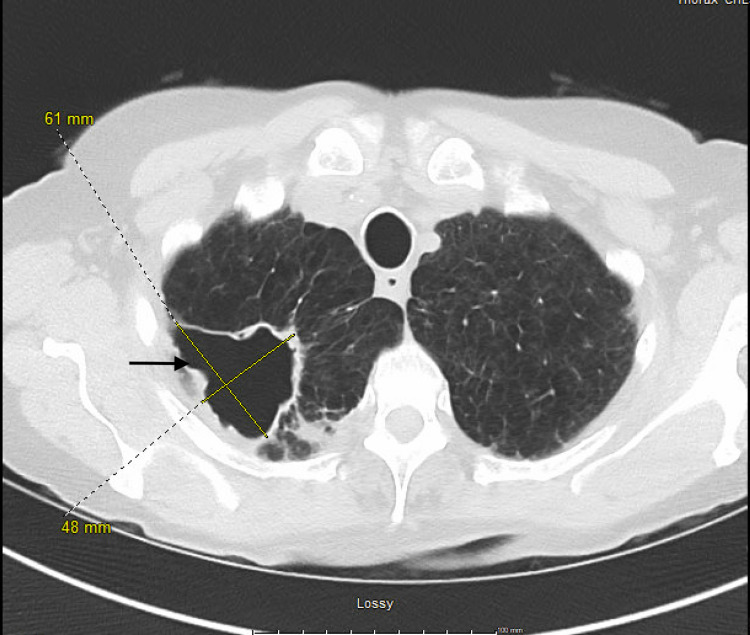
Axial view of follow-up CT chest showing no change in the size of the cavitary lesion with interval clearing of the cavity.
